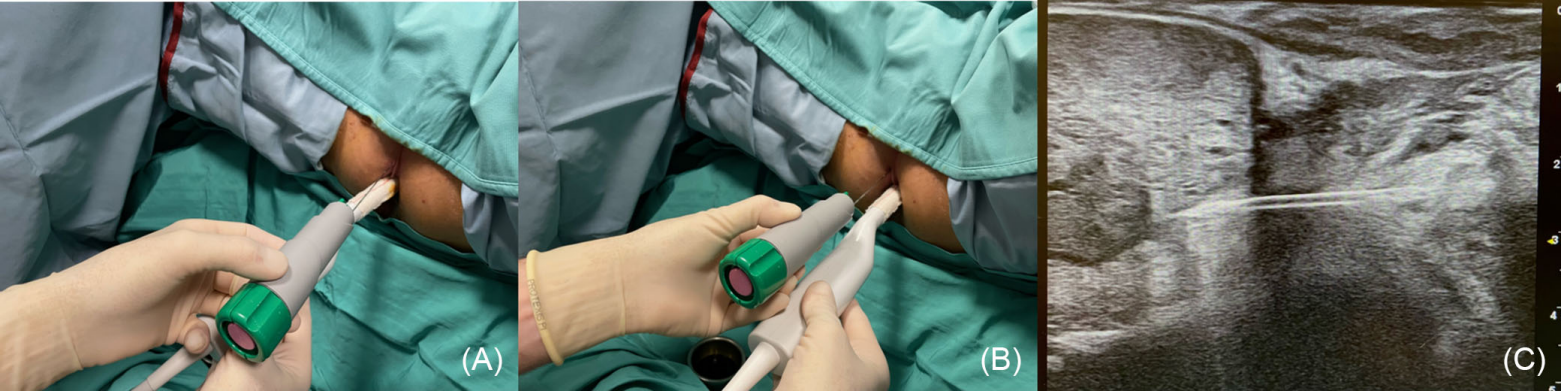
Double‐freehand transperineal prostate biopsy technique. (A) Inadequate needle alignment to the ultrasound probe and needle tract distortion. (B) Adequate needle alignment to the ultrasound probe, with no distortion. (C) Ultrasound view of an appropriately aligned needle in double‐freehand transperineal prostate biopsy.
